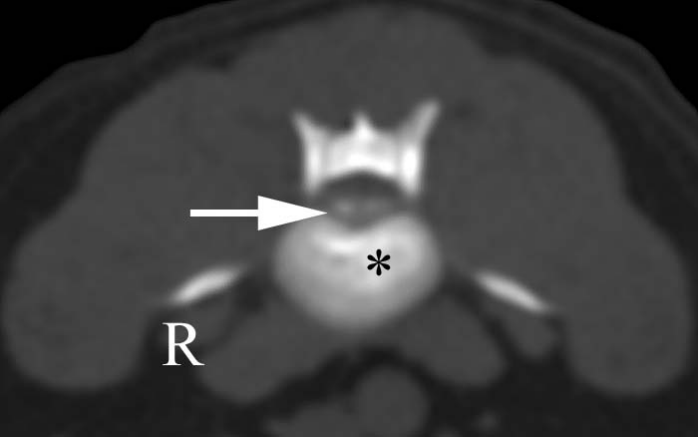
CT scan in bone window through L3-4 showing calcified material (arrow) ventrally in the spinal canal. The spinal cord is compressed. The intervertebral disc is calcified (*). These findings suggest an intervertebral disc extrusion at L3-4 that was confirmed at surgery.
